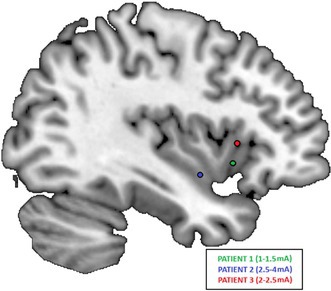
FIGURE 1 Representation of the midway location of the bipolar SEEG electode pair evoking reproducible ecstatic symptoms for the 3 patients, projected onto a standard sagittal T1‐MRI of the MNI atlas brain.
